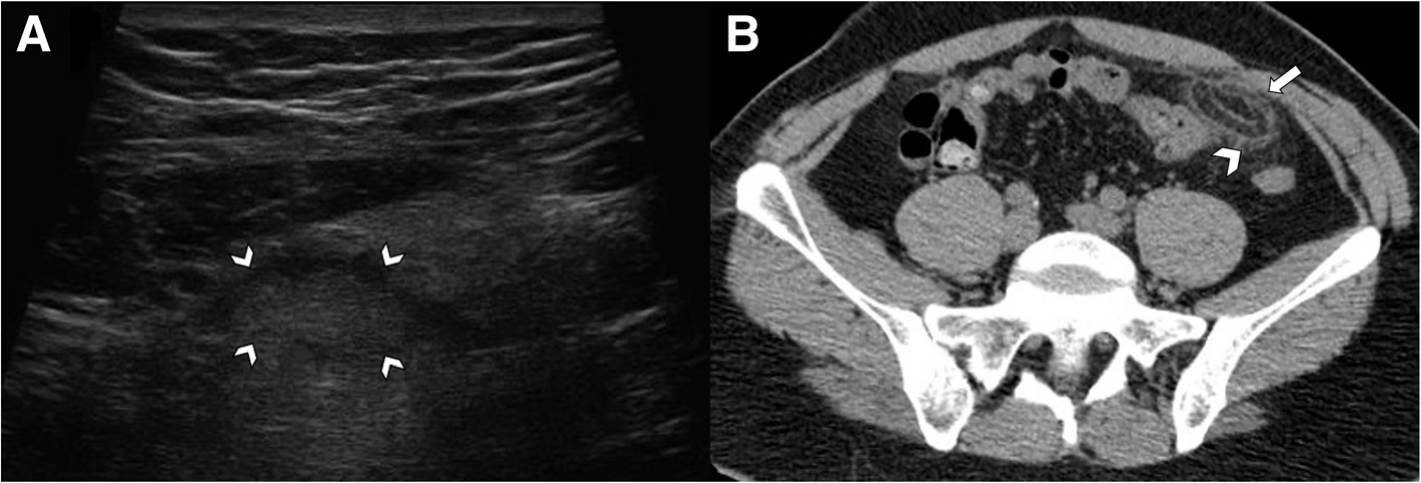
Acute epiploic appendagitis in a 41-year-old man. a Ultrasound transverse scan, at the point of maximum tenderness, shows a well-defined hyperechoic ovoid fat lesion, surrounded by hypoechoic rim (arrowheads), adjacent to the anterior abdominal wall. b Axial non-contrast CT image shows an inflamed fatty ovoid lesion near the sigmoid colon with hyperattenuating ring (arrow) and nearby fat stranding (arrowhead)
